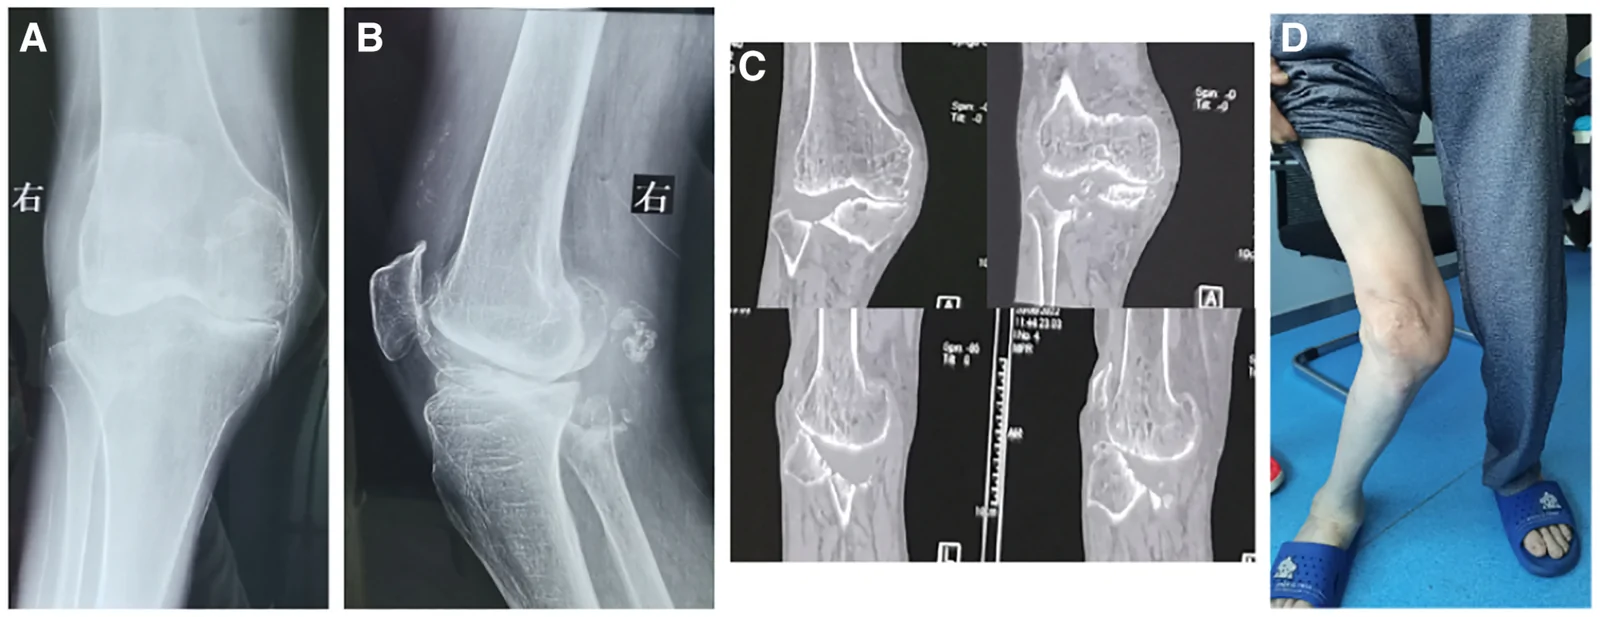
(A) Preoperative anteroposterior view radiograph of the right knee. (B) Preoperative lateral view radiograph of the right knee. (C) Non-contrast CT scan of the right knee joint reveals a bone defect in the lateral tibial plateau. (D) Preoperative anterior view of the patient’s right knee.
